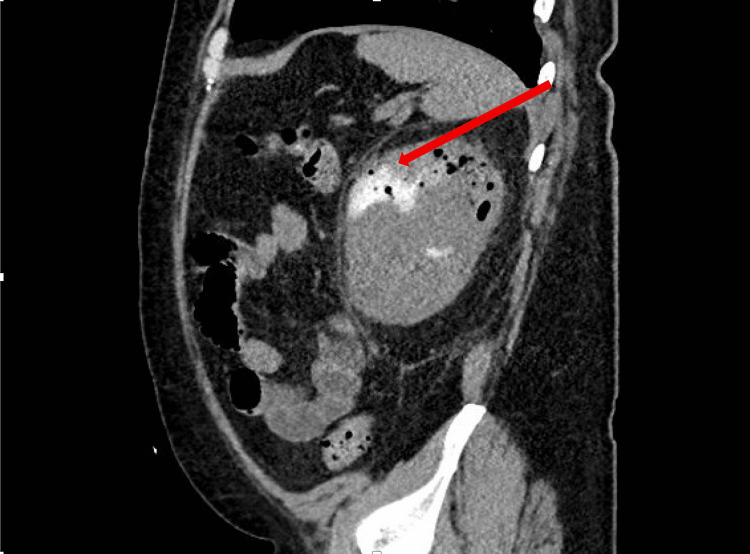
CT scan showing emphysematous pyelonephritis developing into a renal abscess (red arrow)
